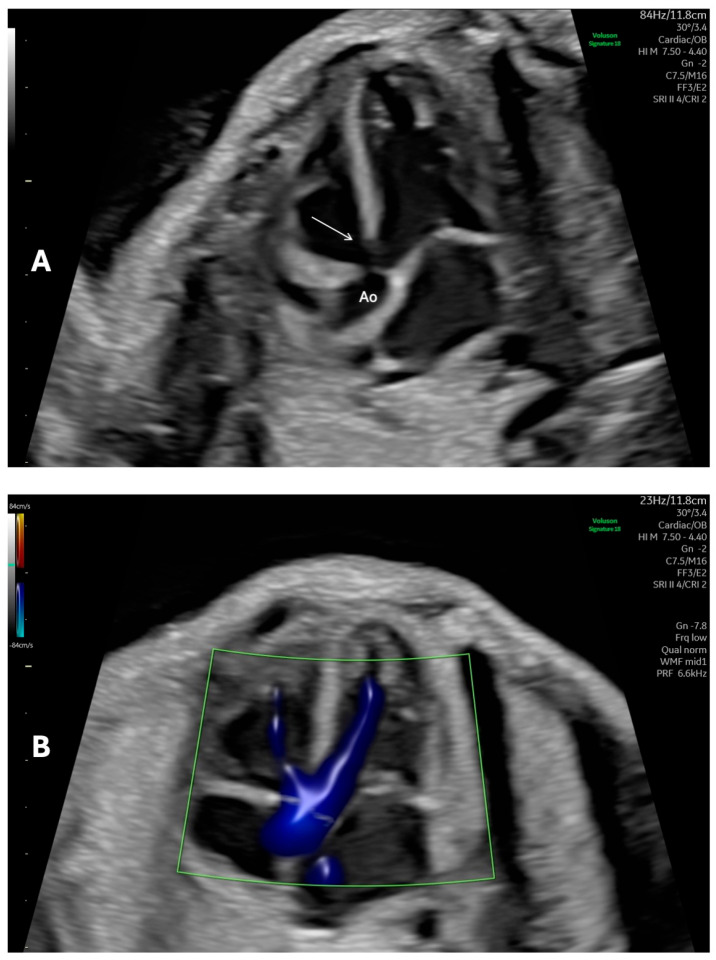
TOF in a Down syndrome fetus of 24 weeks: the aorta (Ao) overrides a complete VSD (arrow) (A). (B) US color Doppler better shows the overriding aorta (blue vessel) and blood flow from both ventricles.
